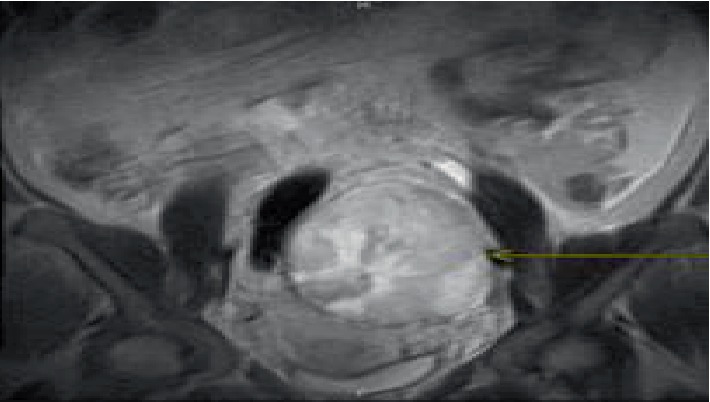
MRI pelvis without contrast coronal view revealed heterogeneous T2 hyperintense left ovarian mass measuring 12 × 9 × 10 cm (outlined by arrow).
